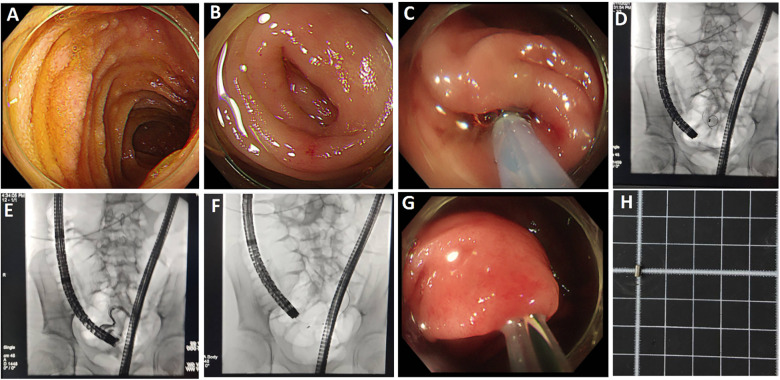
Endoscopic findings during endoscopic retrograde appendiceal foreign body removal assisted by x-ray fluoroscopy (patient 4). (A) Terminal ileum; (B) Appendiceal orifice; (C) Cannulation of appendiceal lumen; (D) Guidewire advancement into appendiceal lumen; (E) Fluoroscopic contrast imaging of appendiceal lumen; (F) Luminal clearance using an extraction balloon; (G) Balloon sweeping through appendiceal lumen; (H) Retrieved cylindrical metal foreign body.
